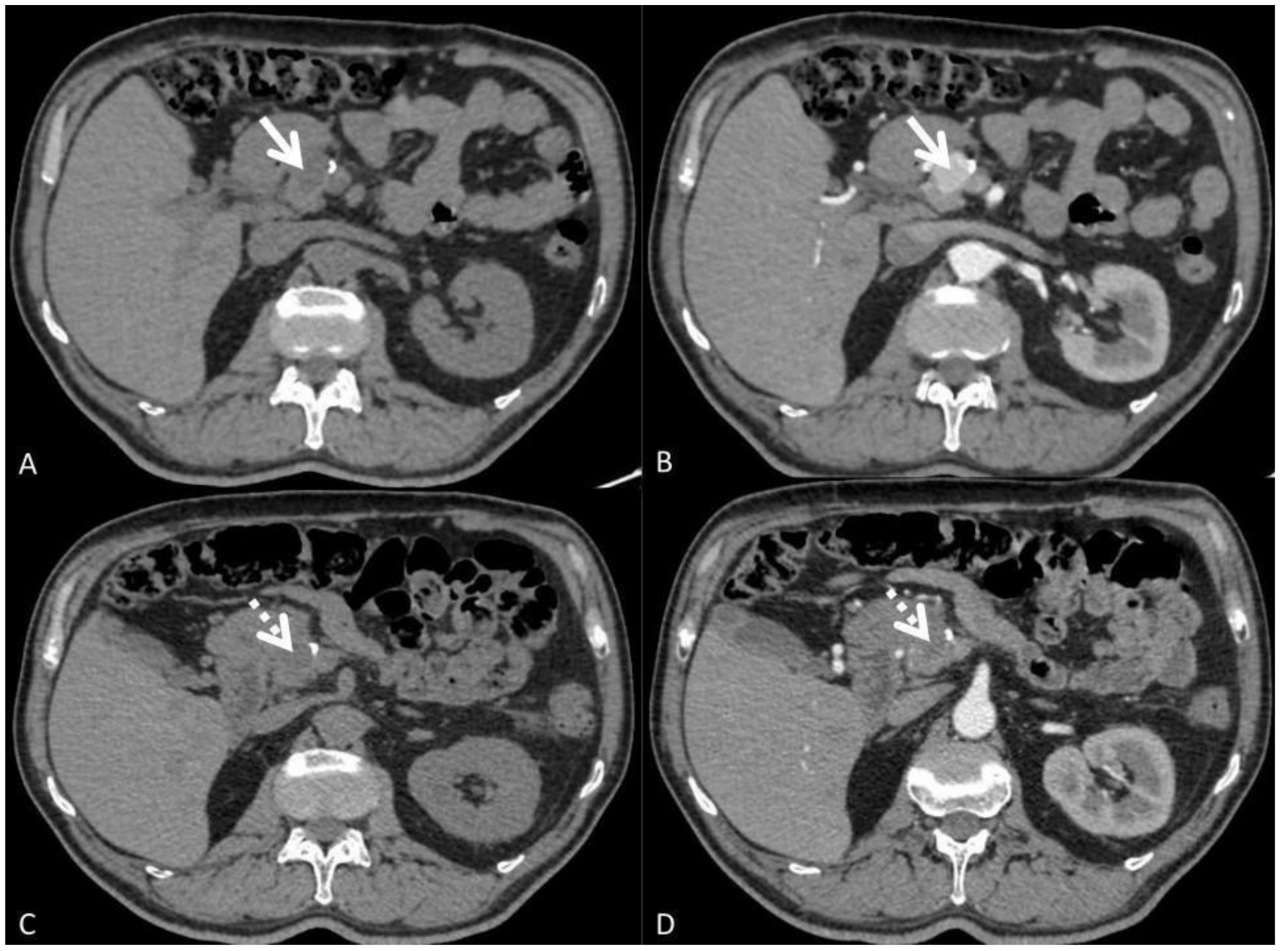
(A–D). Axial non-contrast (A) and arterial phase (B) CT images of the abdomen reveal an avidly enhancing metastasis in the head of the pancreas measuring 2.5x 2.0 cm in diameter (arrow). Follow-up CT images (C, D) obtained after 2 months of treatment demonstrates decreased attenuation of metastatic lesion in the head of the pancreas with minimal size reduction to 2.2 x 1.7 cm (dashed arrow).
